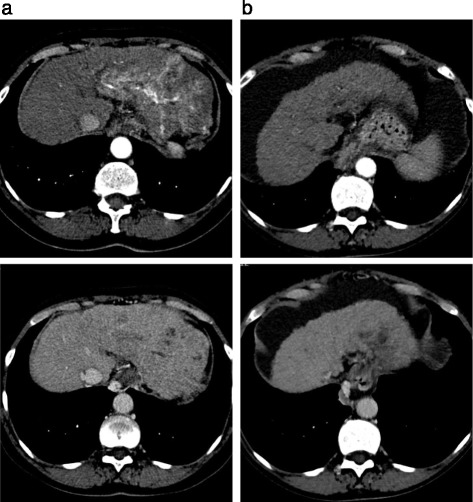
Case 2 response to sorafenib. a. Triple phase CT of the liver 10/06/2013. Primary HCC with arterial phase enhancement (*top panel*) and portal venous washout (*bottom panel*). b. Triple phase CT of the liver 19/12/2014. Primary HCC with no arterial phase enhancement (*top panel*) or portal venous washout (*bottom panel*)
